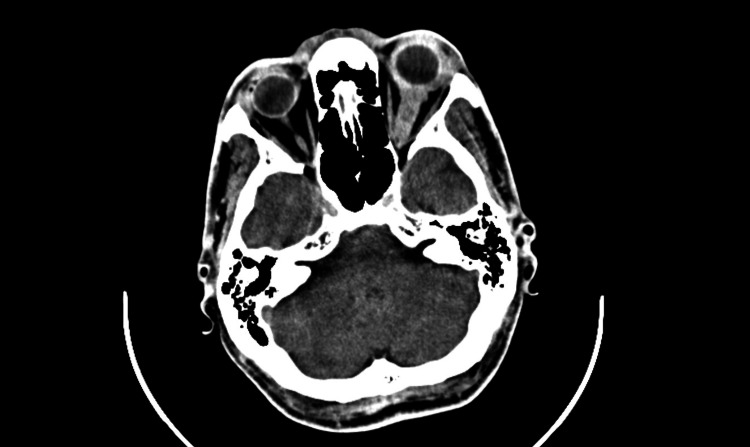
Axial non-contrast computed tomography (CT) scan showing proptosis and structural alterations of the left optic nerve.
